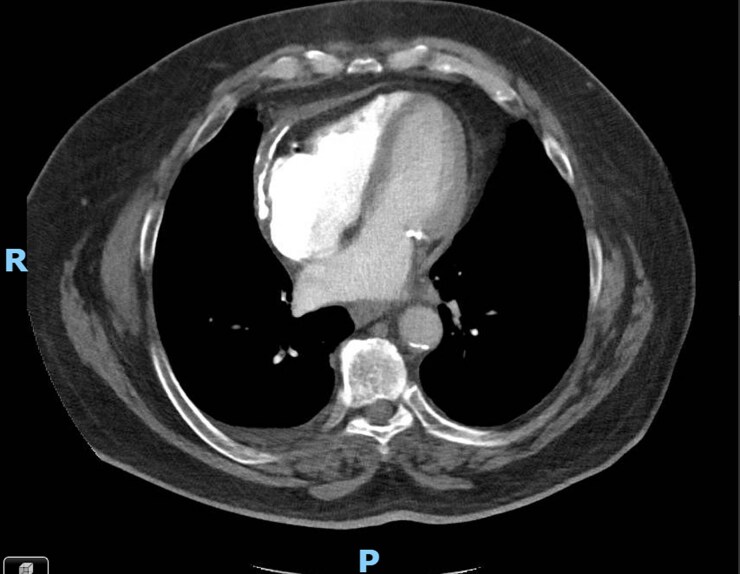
Computed tomography angiography of the chest showing pericardial calcifications overlying the right ventricle.
